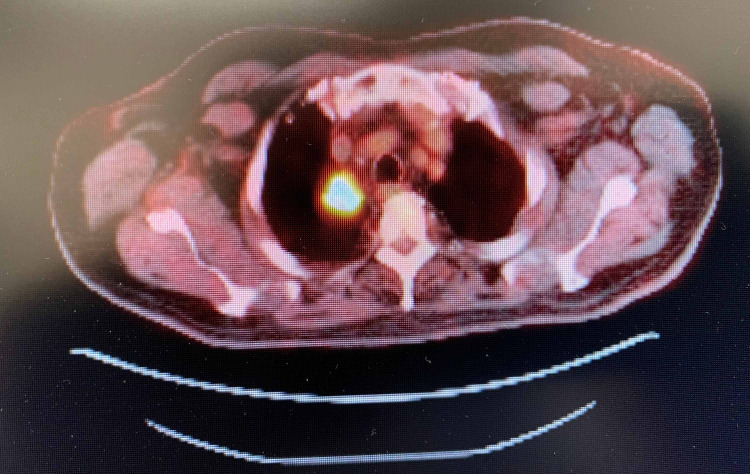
PET scan Hypermetabolic right lung mass abutting the mediastinum, with SUV measuring 14.4 SUV: standardized uptake value; PET: positron emission tomography
